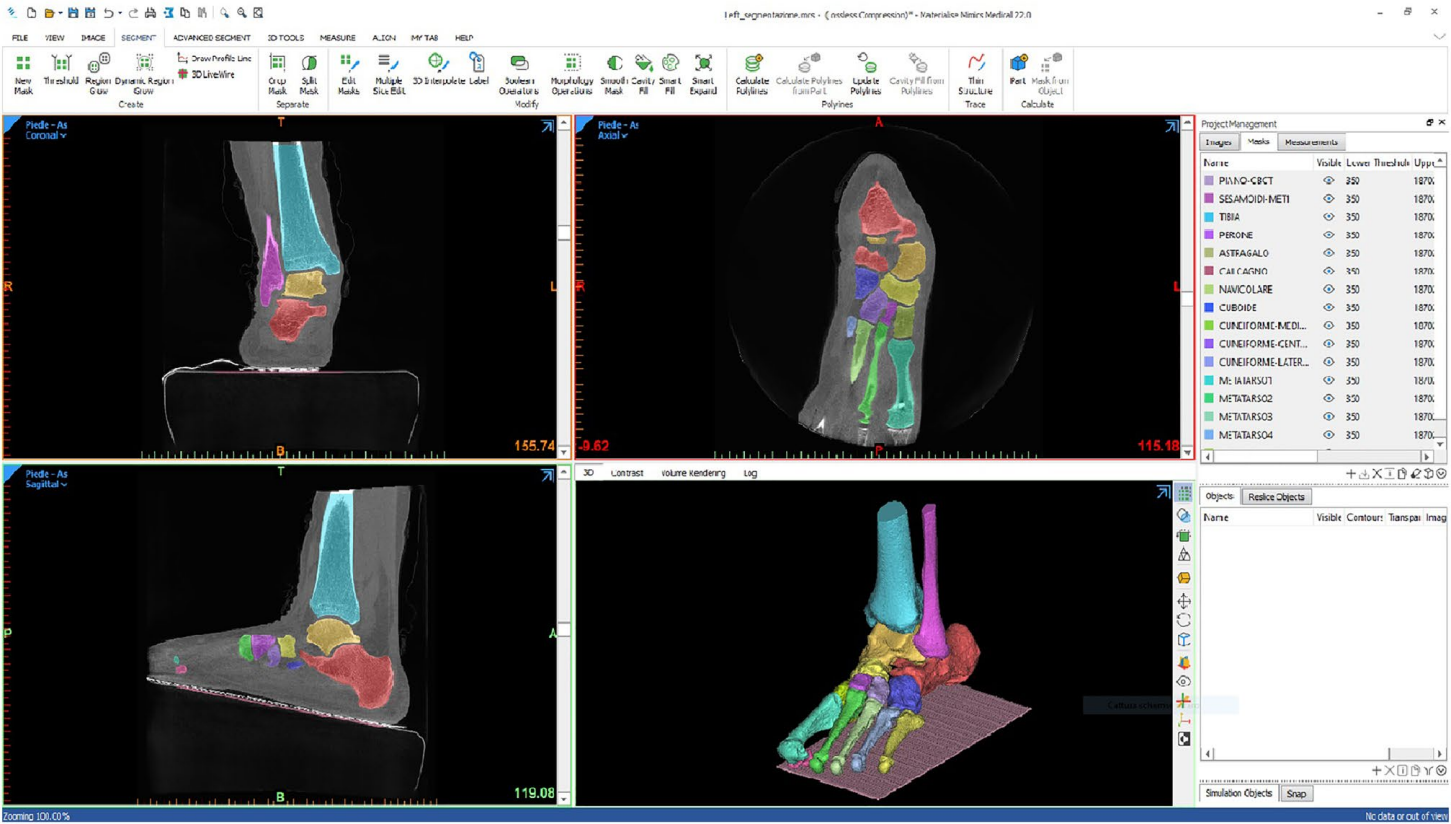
A screenshot from the 3D reconstruction of the foot bones from a CBCT scan by Mimics Innovation Suite (Materialise, Belgium) software. Each bone segment has a different colour, in both the 3D view (bottom-right) and in the three anatomical planar views; in this antero-posterior slice (top-left), the tibia, fibula, calcaneus and talus bone segments are depicted. In the sagittal view (bottom-left), apparently the foot is an inclined ground, but this is accounted for to the inclination of the gantry in the present device, i.e. the foot is on the horizontal ground.
